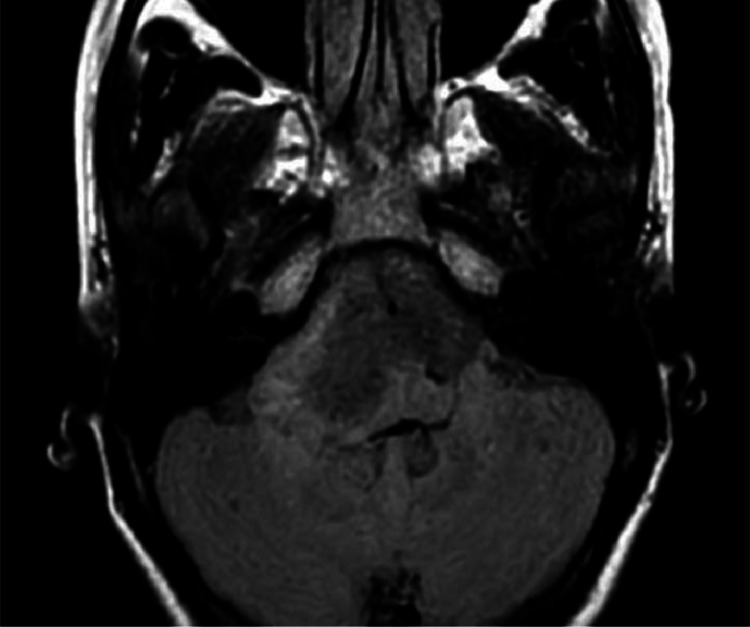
Axial FLAIR image shows a suppression of contents.
